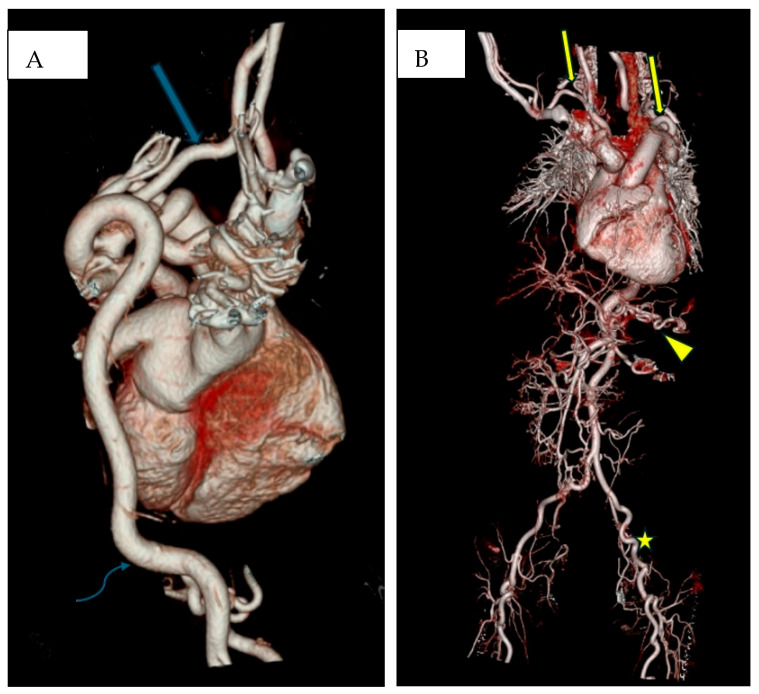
(A): Three-dimensional (3D) rendering from CT angiography shows tortuosity of the aortic arch and its branches (blue arrows) in a patient. (B): 3D rendering from CT angiography shows tortuosity of the aortic arch and its branches (yellow arrow), an elongated abdominal aorta with its coiled branches (yellow arrowhead), along with tortuosity of the lower limb arteries (asterisk) in a patient.
